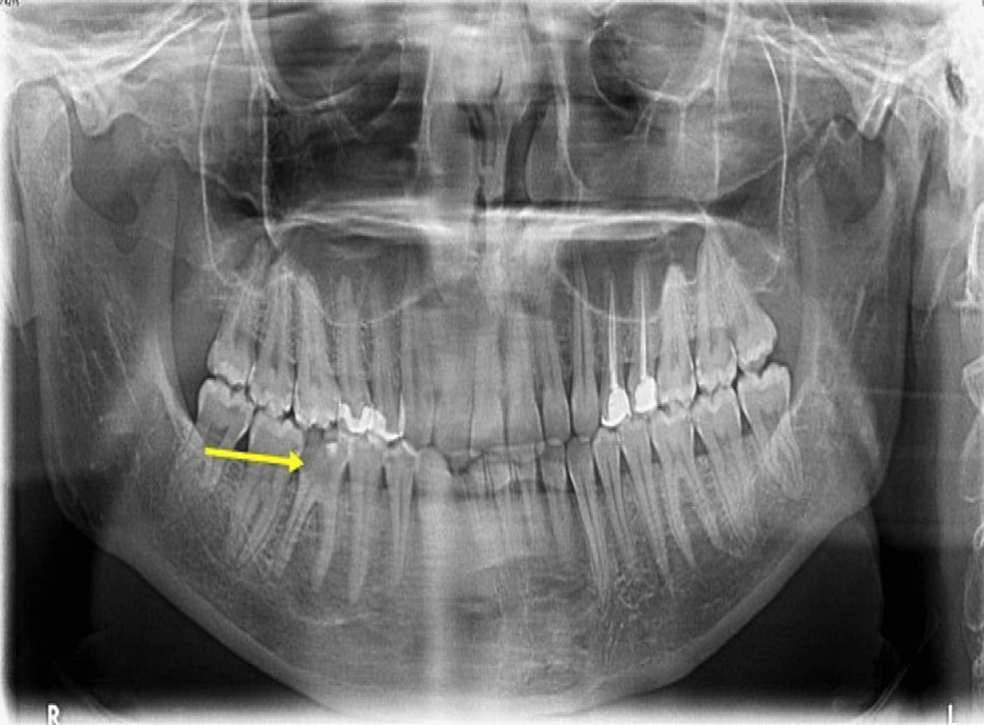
Orthopantomogram image Dental caries were noted on the lower right first molar tooth, but no obvious lucency to the surrounding bone to indicate a bony infection.
